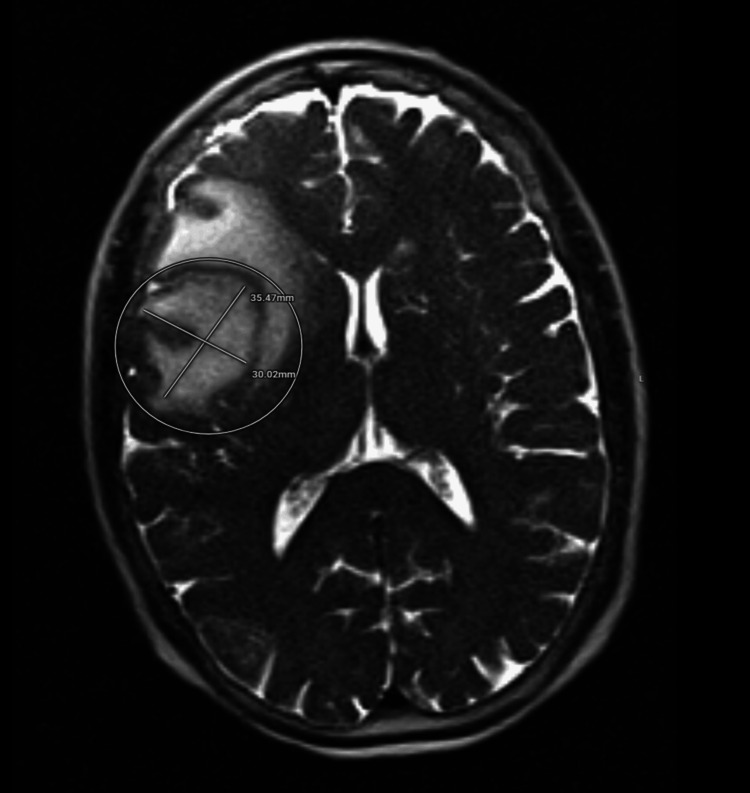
Brain magnetic resonance imaging (MRI), T2-weighted axial image, showing a rim-enhancing mass in the right frontal lobe measuring approximately 3.5 × 3.0 cm. Imaging features include central diffusion restriction within a T2 hypointense rim, most characteristic of a brain abscess, with marked surrounding vasogenic edema.
